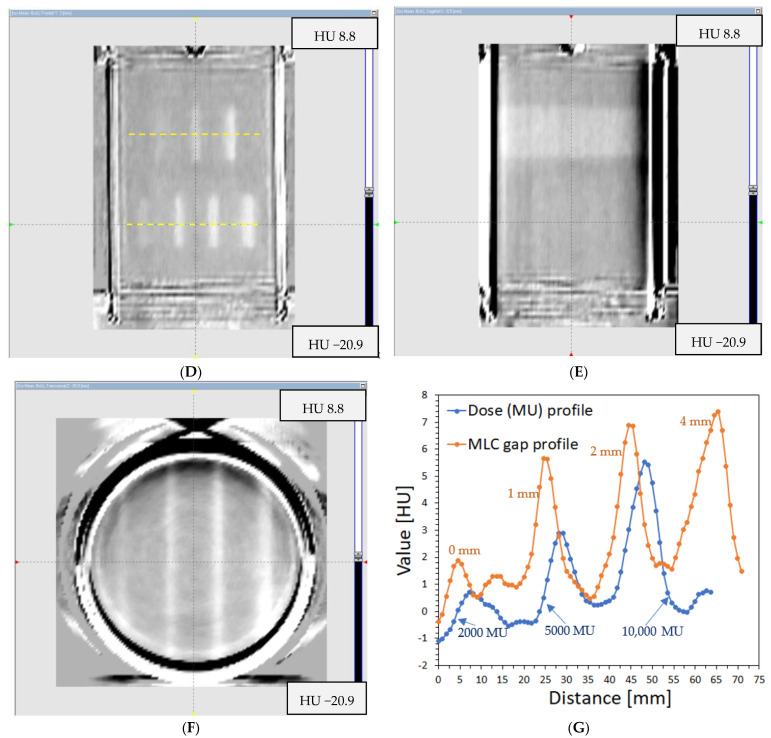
Selection of the radiation field parameters: multi-leaf collimator (MLC) gap and dose (MU) for the determination of the isocenter. The study was performed for PABIG^nx^ in a PH-6-DD2 container (GeVero Co.). In (A–C), there are photographs of the dosimeter after irradiation; (A) is a bottom view in a horizontal position, (B) is a front view of the container in a vertical position and (C) is a side view of the container in a horizontal position. The irradiated regions (B) correspond to different gaps of MLC (closer to the bottom of the container in (B)): 0, 1, 2 and 4 mm (for 10,000 MU), from left to right, and they correspond to 2000, 5000 and 10,000 MU, from left to right (closer to the top of the container in (B)). (D–F) are CT images (iCBCT, TrueBeam, Pelvis): for frontal Y: 3 mm; sagittal X: −0.5 mm; and transversal Z: 23.4 mm, respectively. In (G), profiles across the irradiated regions, corresponding to the irradiated regions for different gaps of MLC and MU, are presented. The yellow dash lines in (D) indicate the positions of the profiles in (G). The profiles were smoothed by applying a mean filter (Kernel mode: 2D, Kernel unit: pixels and Kernel size 5 and 3 for Dose (MU) profile and MLC gap profile, respectively).
